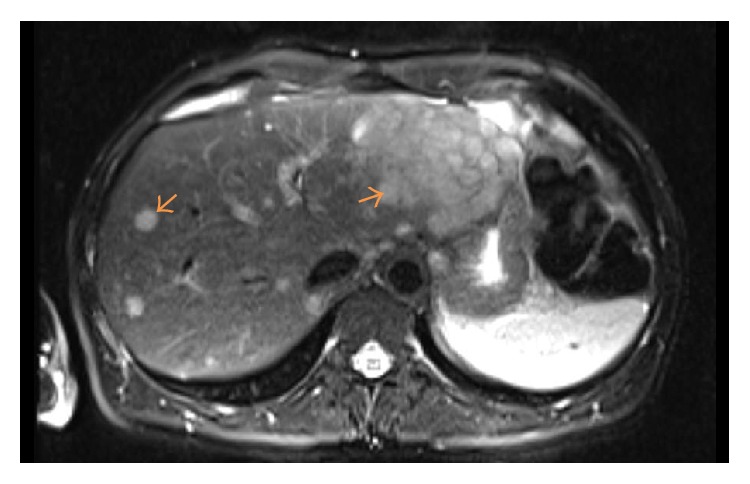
Abdominal MRI imaging scan showing a large dominant mass in the left hepatic lobe along with numerous other smaller hepatic lesions.
